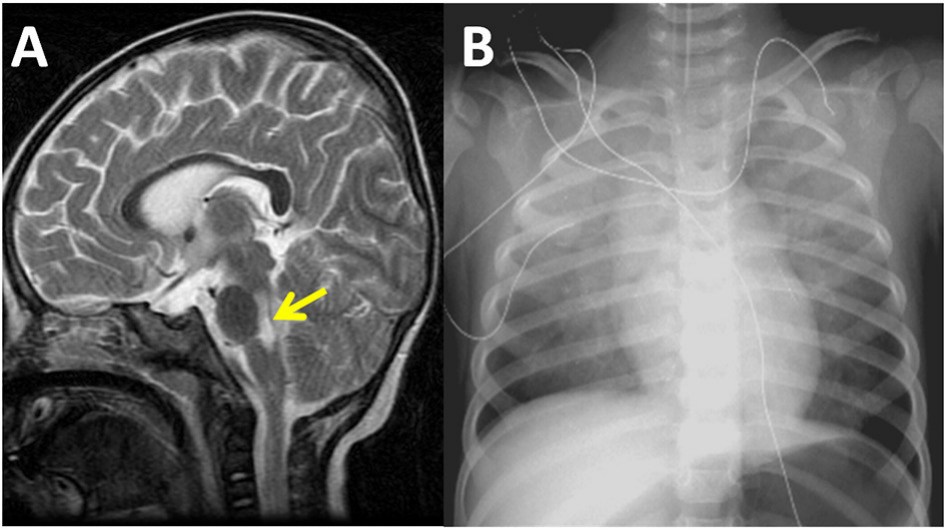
Sagittal magnetic resonance imaging of the brain reveals high signal intensity over the dorsal regions of the brain stem and cervical spinal cord in a 16-month-old patient with severe enterovirus 71 infection and acute heart failure in the year 2001 (A). Chest radiography demonstrates bilateral pulmonary edema and a normal heart size. The ejection fraction of the left ventricle was 27% as measured by echocardiography at this time (B).
